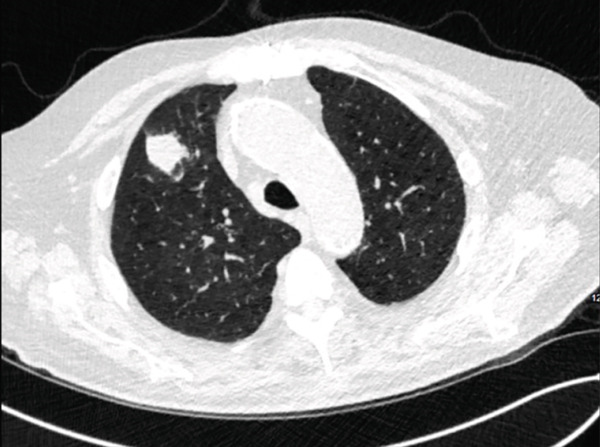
Noncontrast chest CT on April 11, 2023 showing right upper lobe nodules measuring 2.7 × 2.1 cm.
